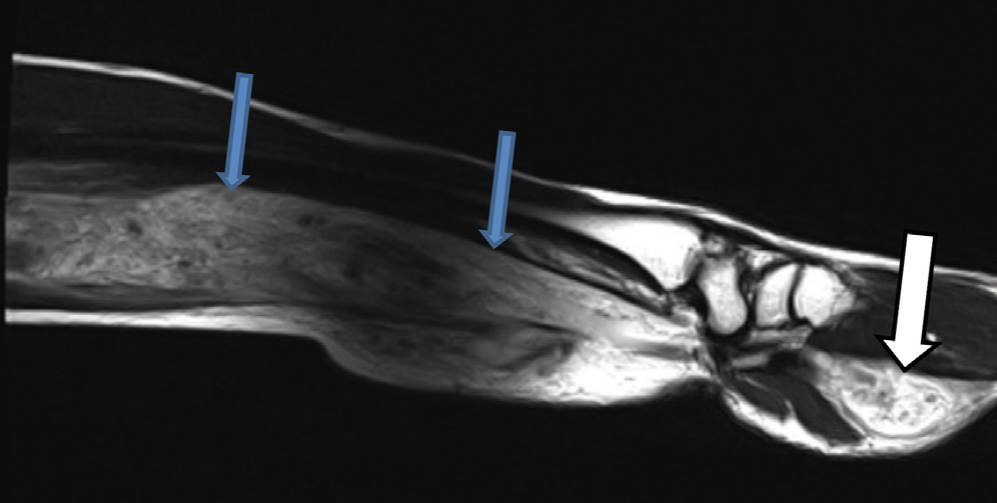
Sagittal T2-weighted magnetic resonance image showing extent of venous malformation of the left forearm (*blue arrows*) and hand (*white arrow*).
